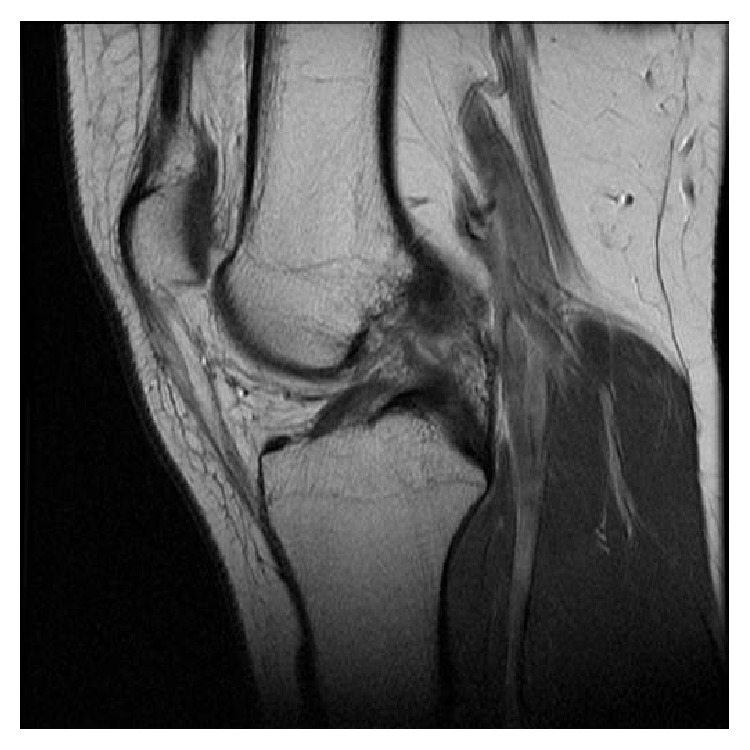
Preoperative sagittal MRI scan of the left knee.
